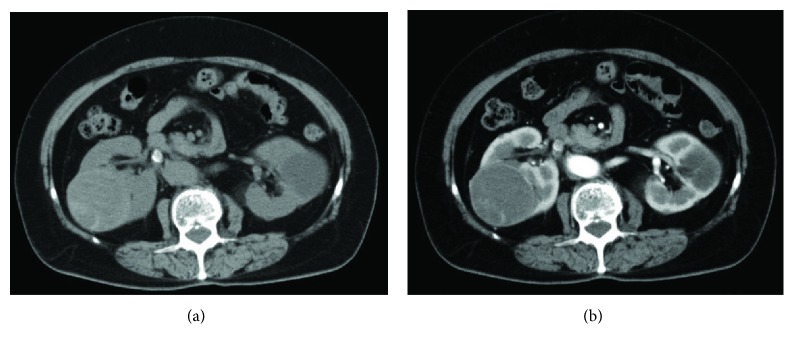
(a) Plain computed tomography image, showing a stone in the right upper urinary tract, but with no indication of the soft protein matrix in the right renal pelvis and calyceal diverticulum, which is radiolucent. (b) Dynamic computed tomography image, showing a complicated cyst, classified as a Bosniak type IIF cyst, arising from the right side of the kidney.
